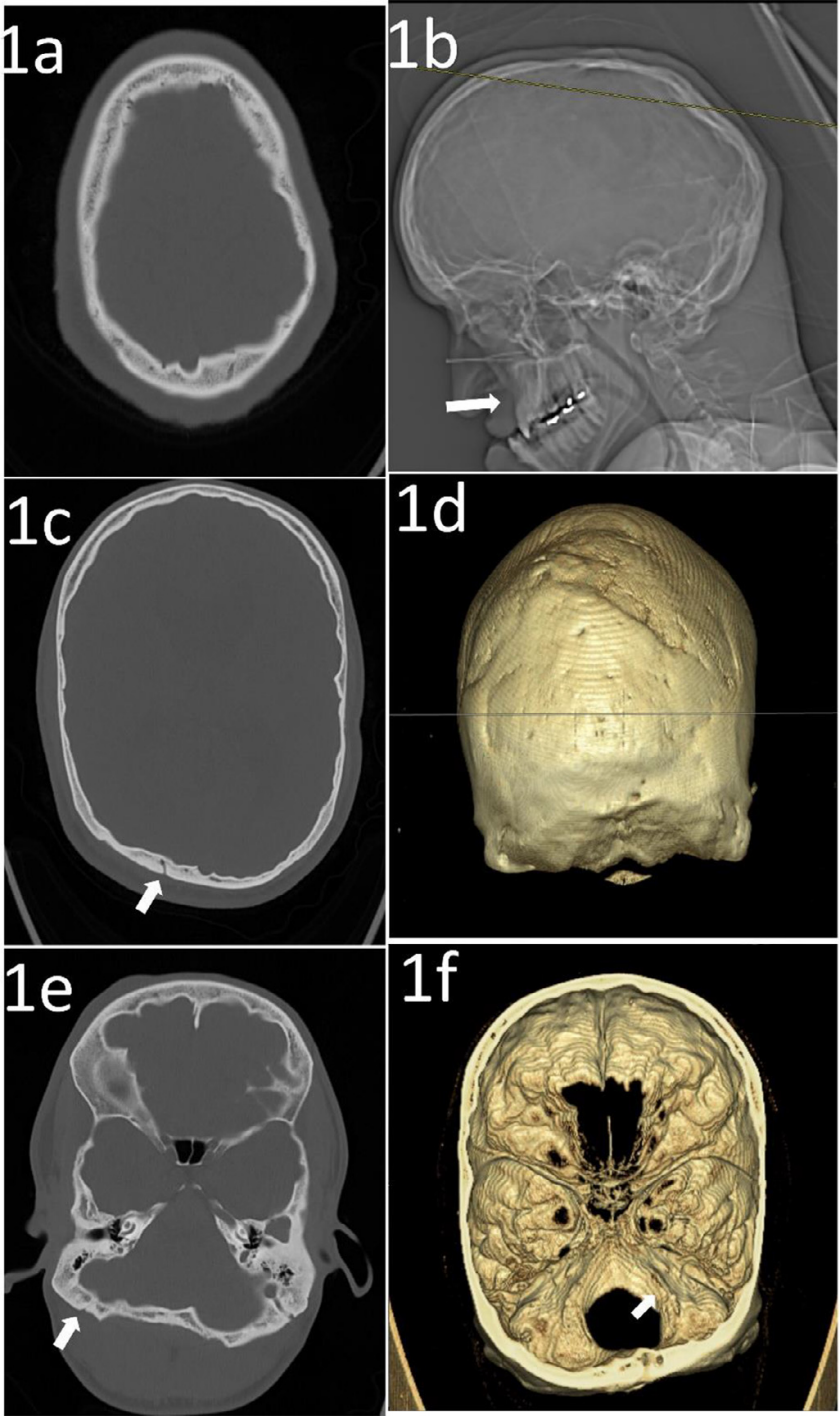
A 21-year- old male with a known Pfeiffer Syndrome presented following a trauma without fractures. Axial brain CT without IV contrast in bone algorithm at multiple levels (1A) through (1E) demonstrates absence of coronal and sagittal sutures. The Lambdoid suture is faintly visualized, white arrows (1C) and (1E). A scout view (1B) demonstrates prognathism due to maxillary hypoplasia. (1D) and (1F) a 3D reconstruction of the skull. (1D) Again, showing the absence of the coronal, sagittal, and lambdoid sutures. (1F) shows an anomalous Jugular foramen.
